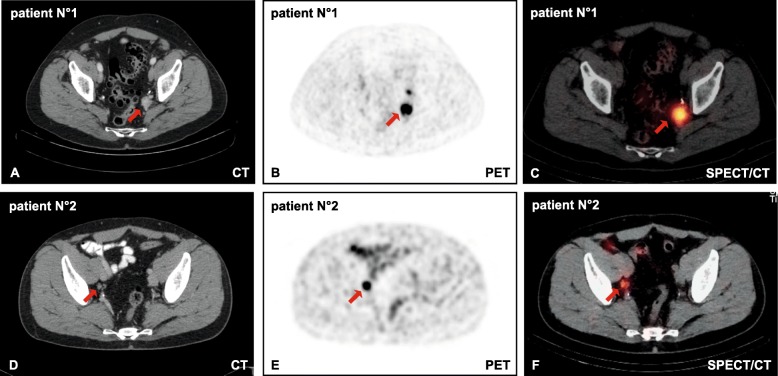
a, d Transversal CT and b, e PET of a PSMA-PET/CT with from two different representative patients no. 1 and no. 2 with suspected lymph node metastases (LNM) prior to lymphadenectomy. c, f Preoperative (48 h preoperatively) transversal SPECT of the same patient. Suspected LNMs are indicated by a red arrow
